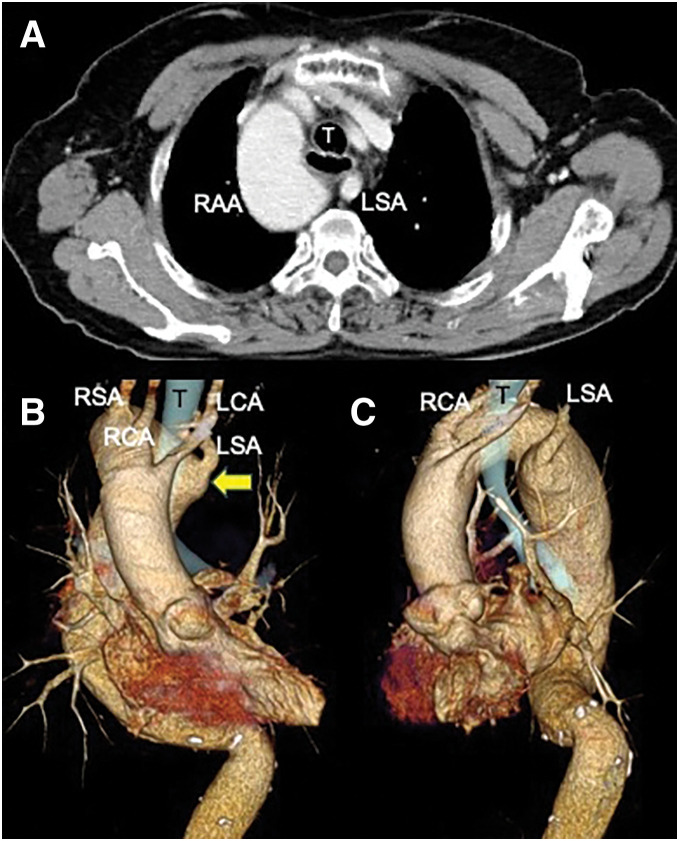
CT images. (A) CT showed esophageal wall thickening in the upper thoracic esophagus without lymph node metastasis. The aberrant LSA courses dorsal to the esophagus, which was surrounded by the RAA, its branch, and the T. (B) An anterior view of 3D-CT also showed an RAA with branching in the following order: LCA, RCA, RSA, and aberrant LSA. A KD was present at the origin of the aberrant LSA (arrow). (C) 3D-CT in the left lateral view. KD, Kommerell’s diverticulum; LCA, left common carotid artery; LSA, left subclavian artery; RAA, right aortic arch; RCA, right common carotid artery; RSA, right subclavian artery; T, trachea
